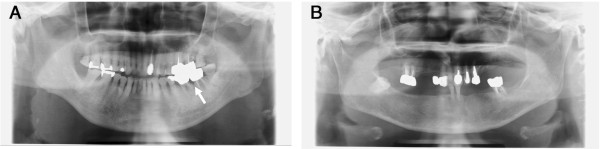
Periapical radiogram. Representative periapical radiogram findings of non-diabetic (A) and diabetic (B) nephropathy patients are shown. Mild horizontal and vertical alveolar bone resorption was found in a non-diabetic (A) nephropathy patient. Moderate horizontal alveolar bone resorption and a lot of missing teeth were found in a diabetic (B) nephropathy patient. An arrow indicates vertical alveolar bone resorption.
